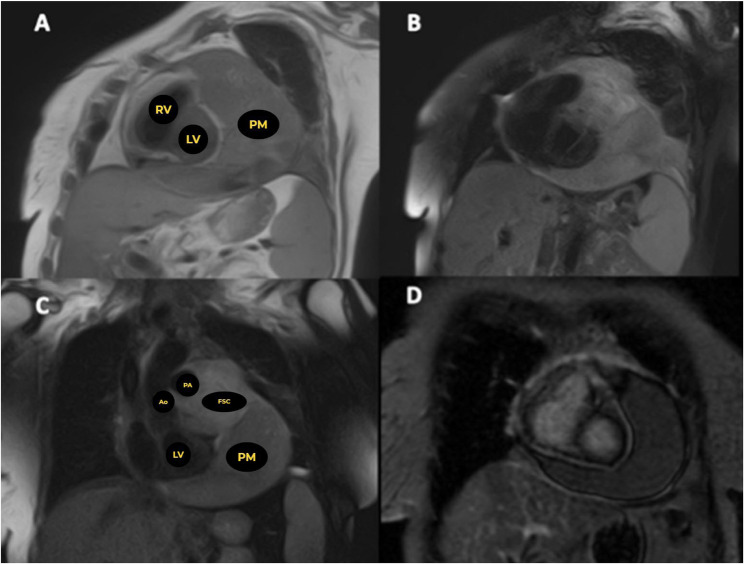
Cardiac Magnetic Resonance Imaging. A and B: T1 and T2-weighted sequences showing a voluminous, circumferential, and heterogeneous Pericardial mass (PM) associated with a large posterior hemopericardium. C: Short-axis T2-weighted sequence with fat suppression (STIR) revealing a heterogeneous mass with a superior Fatty component (FSC) partially encasing the Pulmonary artery (PA) and the Ascending aorta (Ao). D: T1-weighted sequence with Late gadolinium enhancement (LGE) demonstrating intense peripheral enhancement followed by progressive heterogeneous central enhancement, supporting the diagnosis of a malignant mesenchymal tumor. RV = Right ventricle, LV = Left ventricle, PM = Pericardial mass, Ao = Aorta, PA = Pulmonary artery PM = pericardial mass FSC = Fatty solid component of pericardial mass
